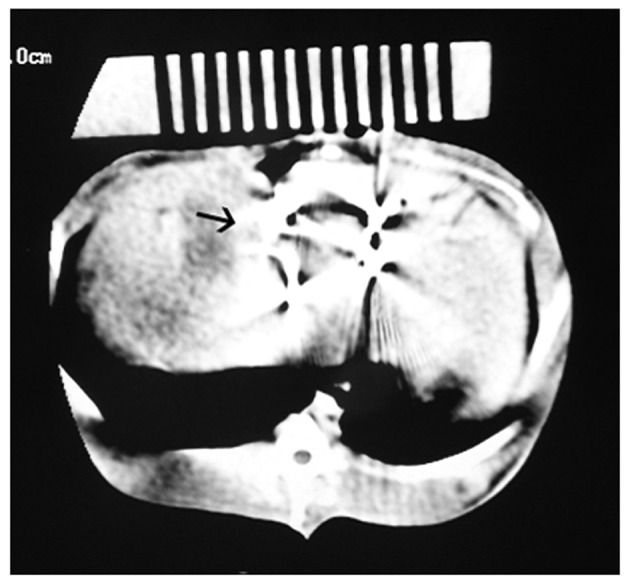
Computed tomography (CT) plain scanning images demonstrate that the ^125^I seed seeds (black arrow) were implanted around the tumor during the CT-guided ^125^I seed brachytherapy (0.5 mCi, range dose, 60 Gy).
